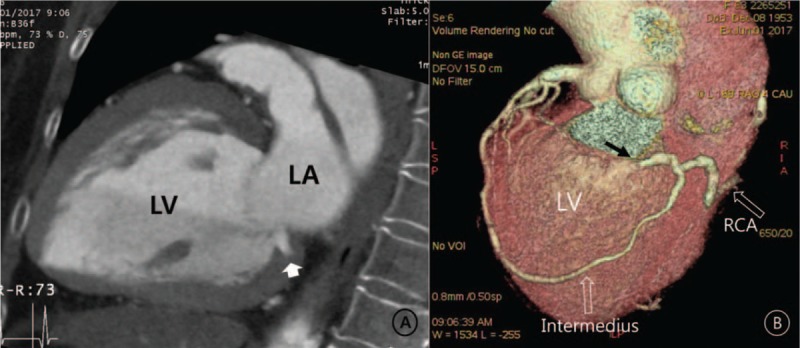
The cardiac computed tomography proved the fistula (short arrow) penetrated the basal inferior wall of the LV (A). The 3-dimensional volume-rendering image clearly displayed the courses of the intermedius artery and RCA. A black arrow: entering point (B). LV = left ventricle, RCA = right coronary artery.
